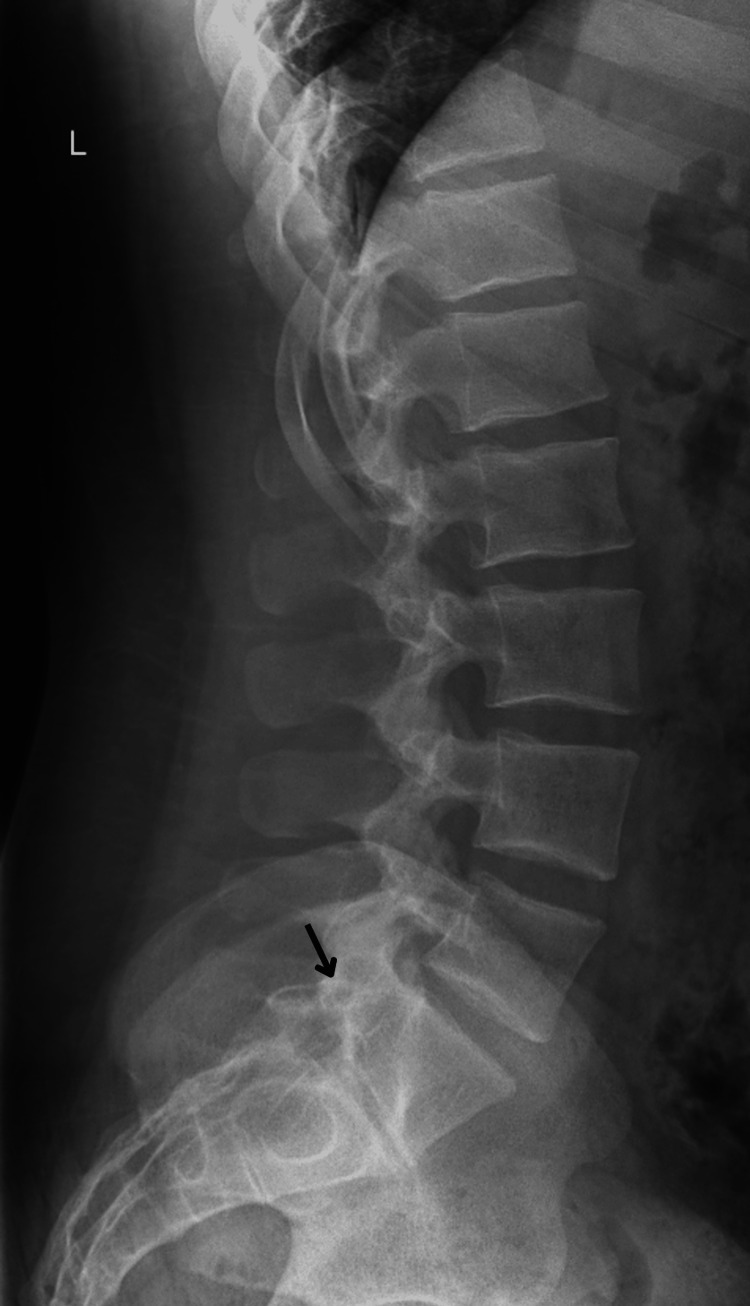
Lateral lumbar spine radiograph with transitional transverse process of the fifth lumbar vertebrae (indicated with black arrow).
